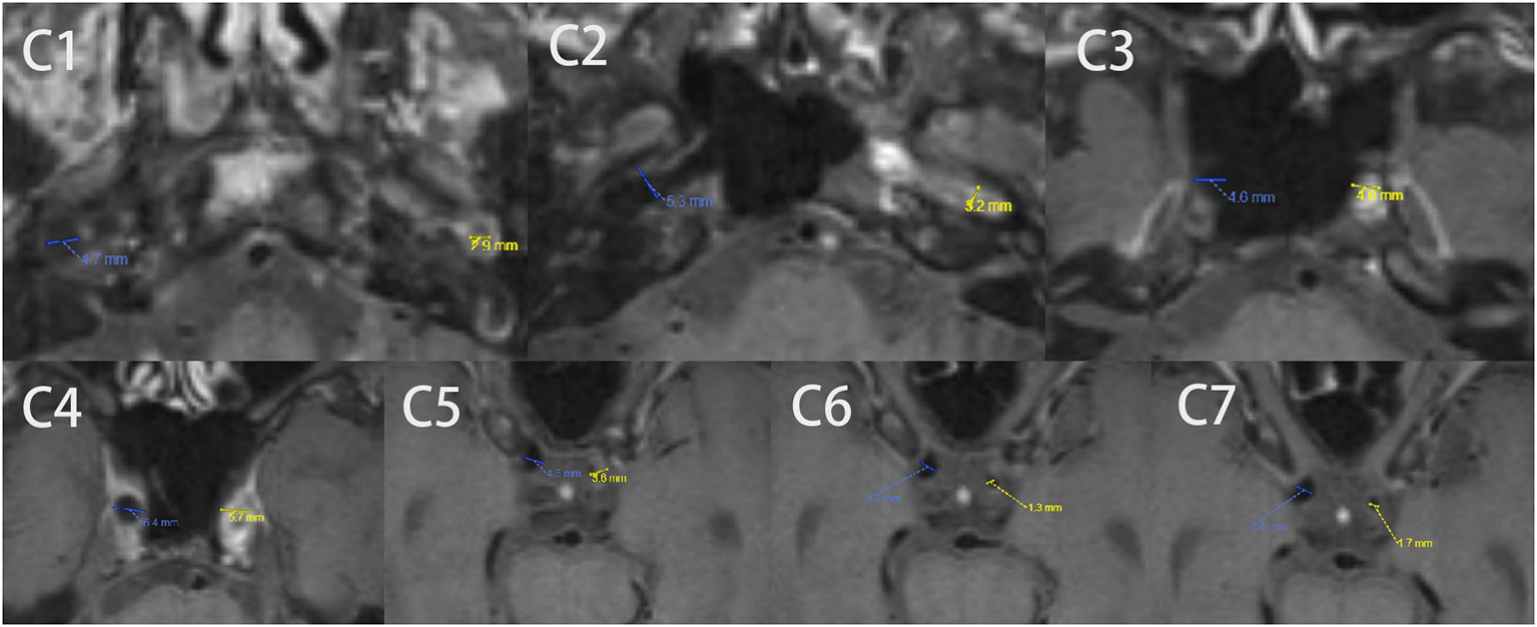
A typical example of C1-C7 diameter measurements in the axial image by HRVWI of ICAO patients. High-resolution vessel wall magnetic resonance imaging (HRVWI) examination with contrast before the procedure. C0, common carotid artery; C1, cervical; C2, petrous; C3, lacerum; C4, cavernous; C5, clinoidal; C6, ophthalmic; and C7, communicating segment of internal carotid artery.
